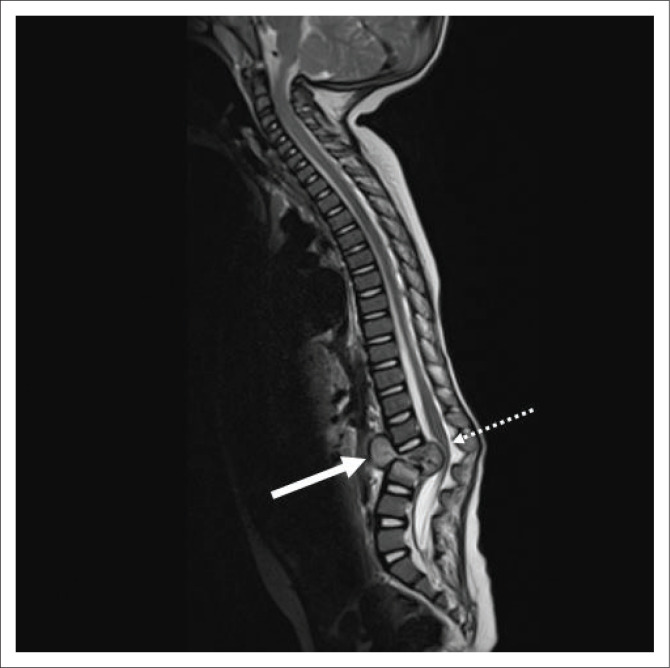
A 3-year-old girl, human immunodeficiency virus negative, presented with weight loss, acute onset of back pain in the lumbar region and refusal to walk. Chest radiograph findings were suggestive of pulmonary tuberculosis. Sagittal T2-weighted magnetic resonance imaging of the spine demonstrates near-complete destruction of the L2 vertebral body with gibbus formation at this site and T2W hyperintense subligamentous abscess (solid white arrow) extending from levels L1 to L3, with compounded compression of the conus medullaris and cauda equina nerve roots (white arrow with dotted line). Tuberculosis was confirmed on tissue GeneXpert.
